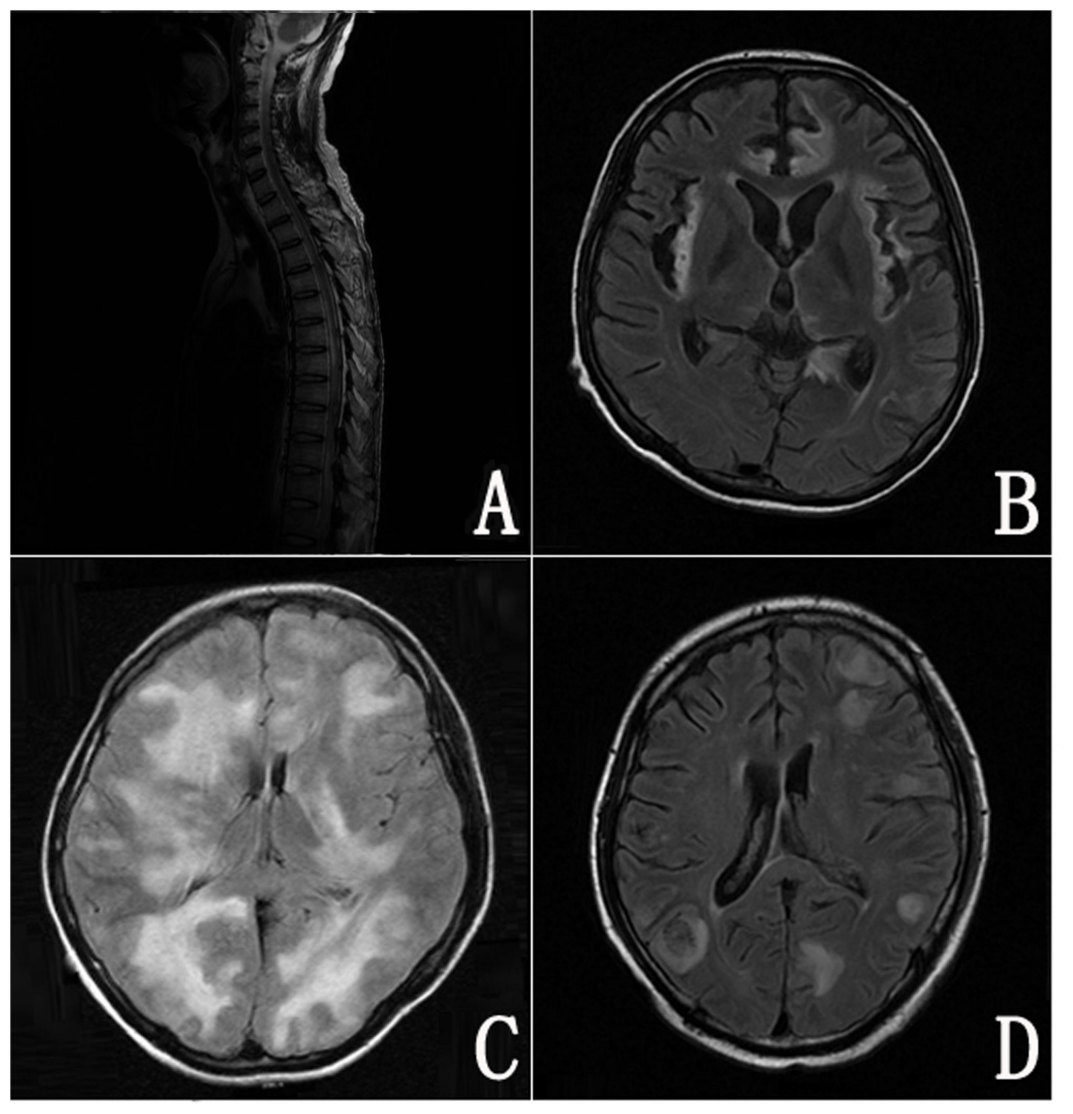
MRI of patients with herpesvirus-associated CNS diseases. A: T2-weighted imaging of a patient with EBV and CMV co-infection reveals disseminate abnormality signals changes in spinal cord; B: MRI of a patient with HSV-1 encephalitis shows bilateral and symmetric distribution of hyperintense lesions involving the frontal and temporal lobes, insula and splenium of corpus callosum on FLAIR image; C: Large patchy areas of increased signals are seen in bilateral white matter under the cortex on FLAIR image in a patients with EBV encephalitis; D: Multiple cloudlike lesions adjacent to pallium and paraventricular lesions are hyperintense on FLAIR image in a patient with EBV-associated CNS-PTLD.
